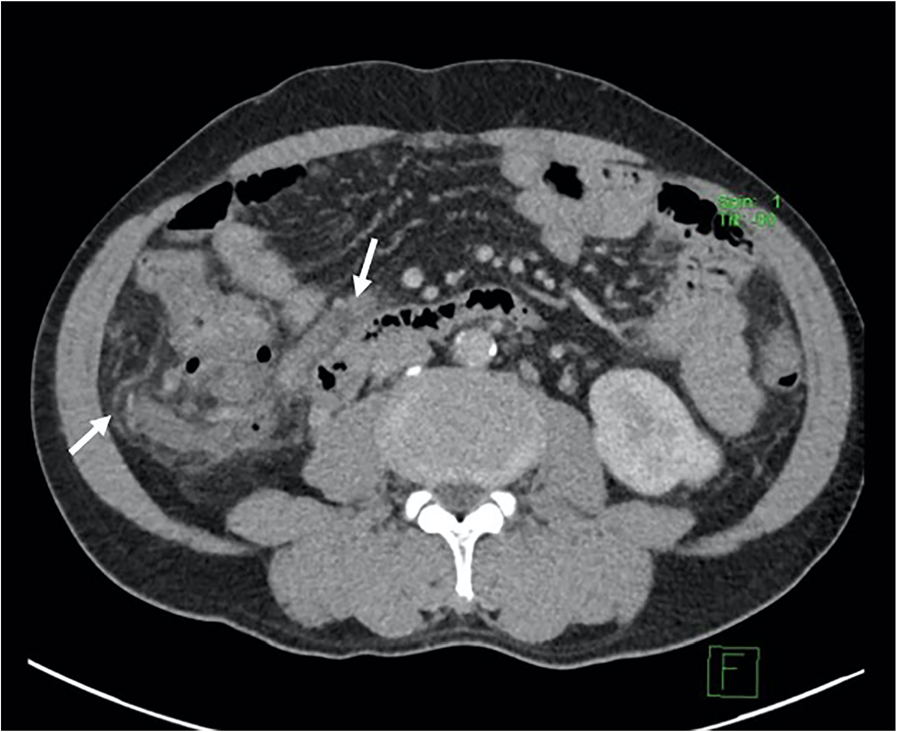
Follow-up computed tomography post-BRTO 2 months later in portal venous phase showing absence of variceal opacification compatible with variceal occlusion (white arrows). There was no evidence of colonic ischaemia
